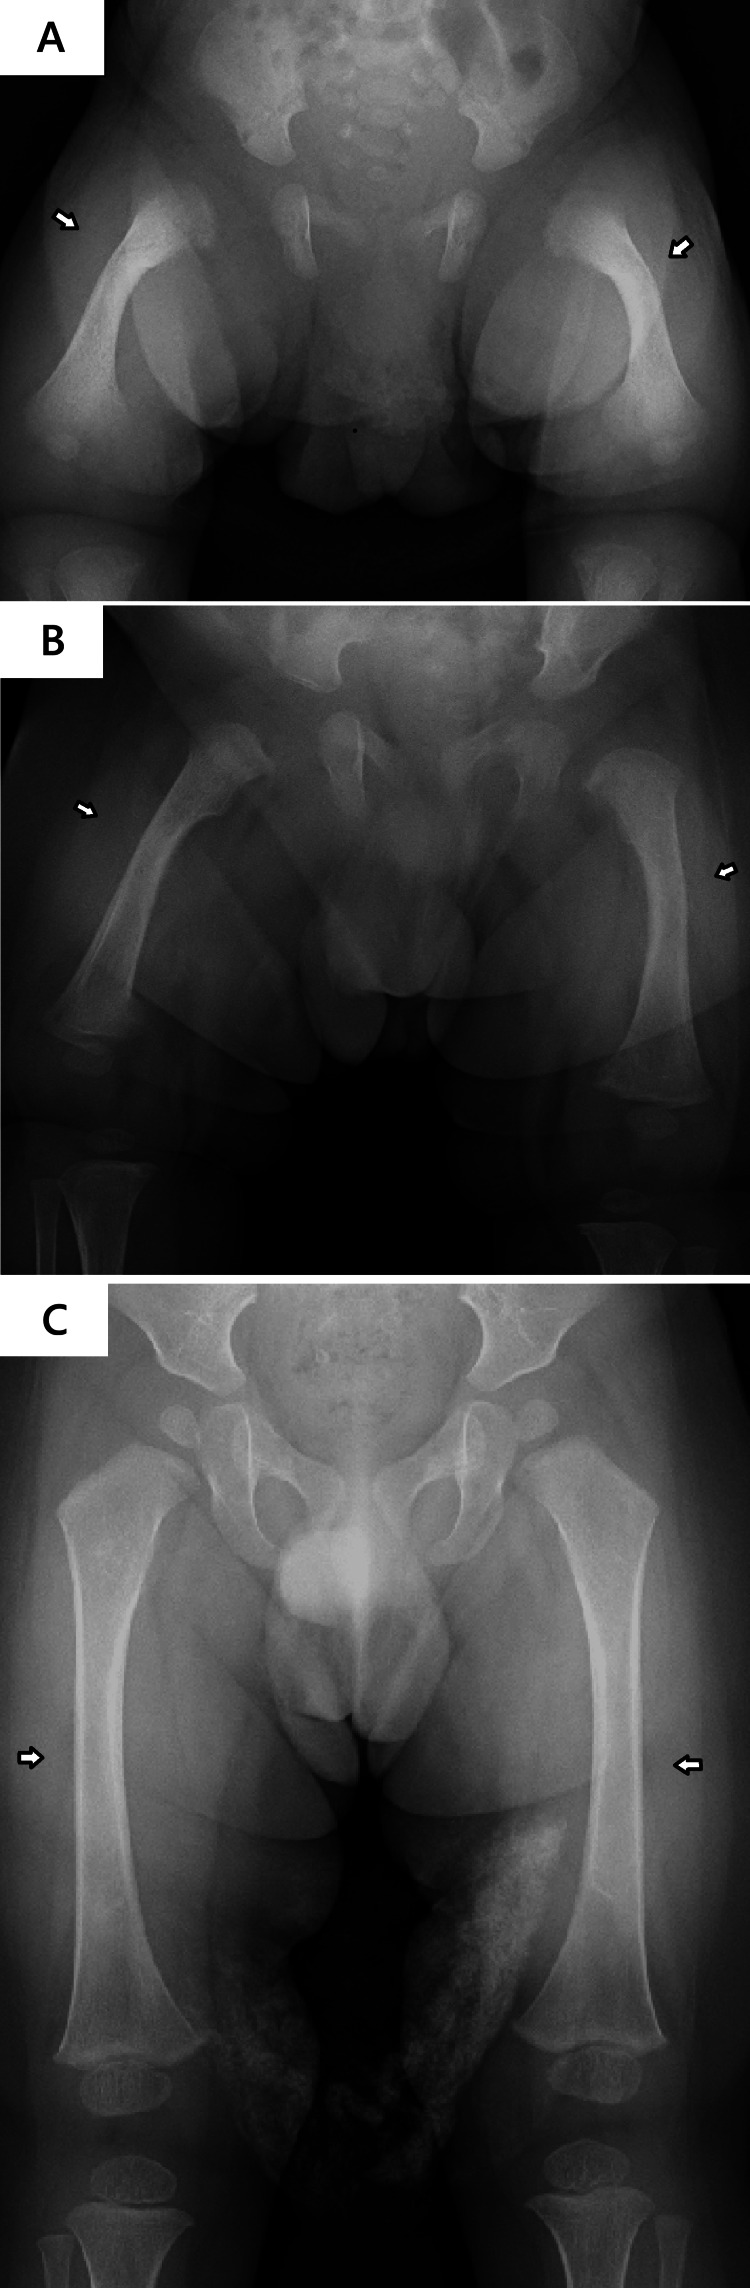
Serial radiographs demonstrating spontaneous resolution of femoral bowing. (A) Birth: Bilateral femoral bowing. Note the absence of rachitic features such as metaphyseal fraying or cupping, which are characteristic of HPP. (B) Two months: Progressive remodeling of femoral bowing. (C) 14 months: Near-normal femoral morphology. HPP: hypophosphatasia.
